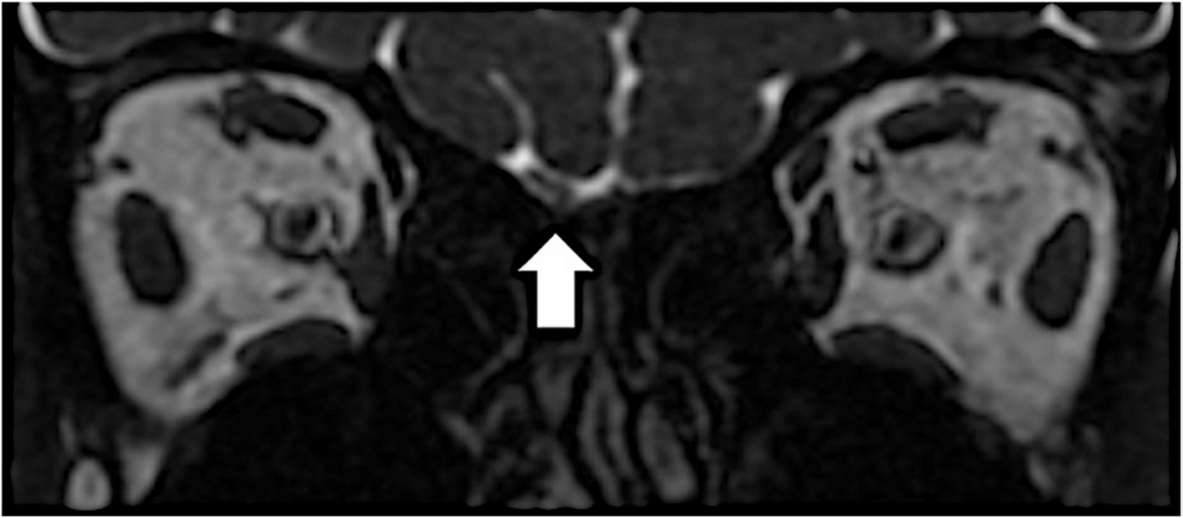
Olfactory bulb atrophy in MRI
